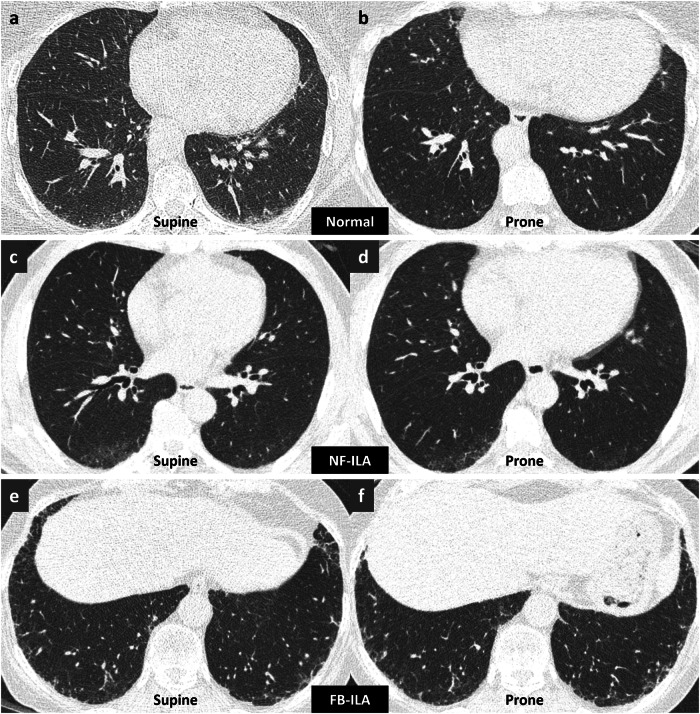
Chest CT images of exemplary cases of a normal subject (a, b), a patient with non-fibrotic ILA (c, d), and a patient with fibrotic ILA (e, f). Subtle ground-glass opacities in the posterior subpleural portion are seen on (a) supine CT and were initially evaluated as positive for ILA by the majority of readers using supine CT alone. The lesions disappeared on (b) prone CT, indicating dependent atelectasis. Subtle ground-glass opacities in the posterior subpleural portion are seen on (c) supine CT and (d) persist in prone CT, indicating non-fibrotic ILA. Mild reticular opacities with traction bronchiolectasis in the subpleural portion are noted in both (e) supine and (f) prone CTs. The majority of readers identified fibrotic ILAs in supine CT alone and supine-prone CTs
